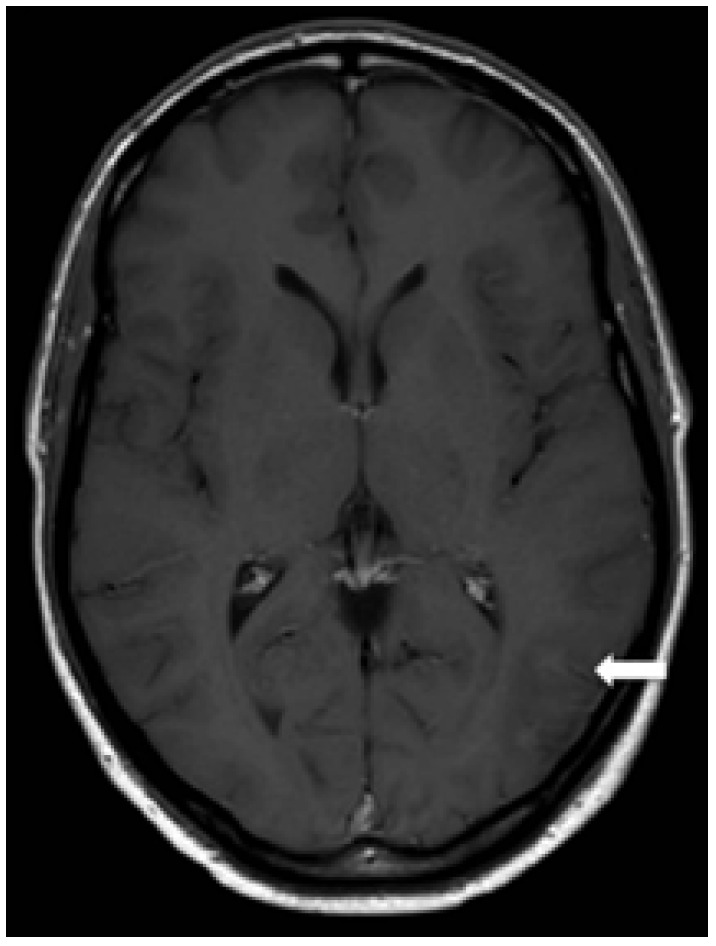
T1-weighted image post-contrast reveals cortical-subcortical hypointensity in left temporal lobe, without enhancement (white arrow).
